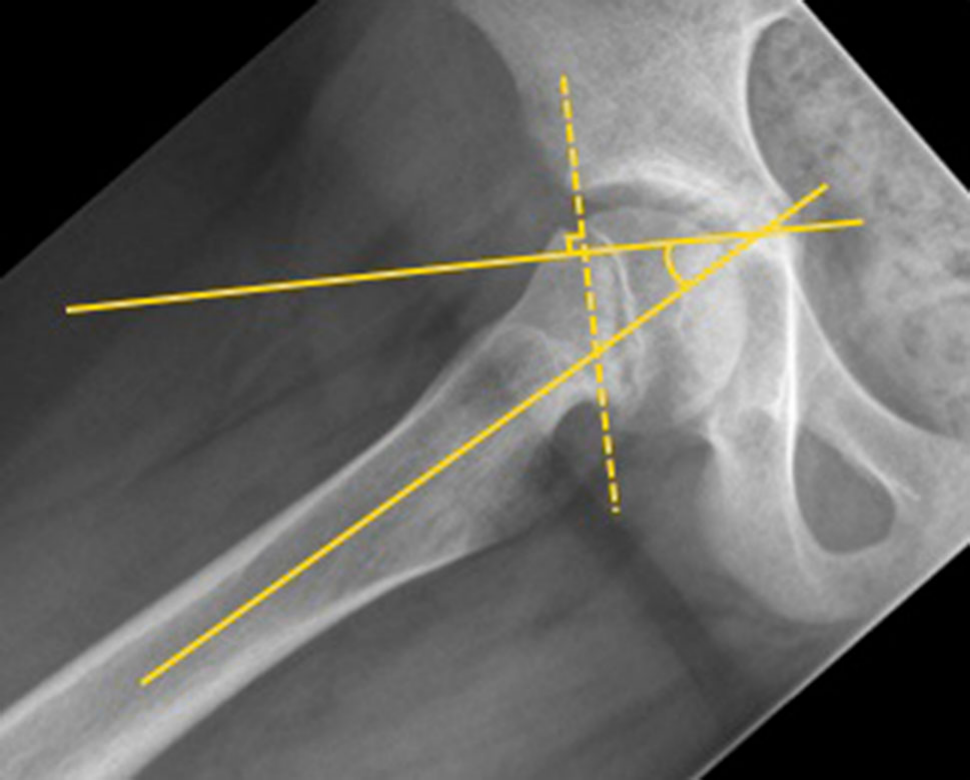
Measurement for head–shaft angle on conventional radiograph (patient no. 5) [16]
